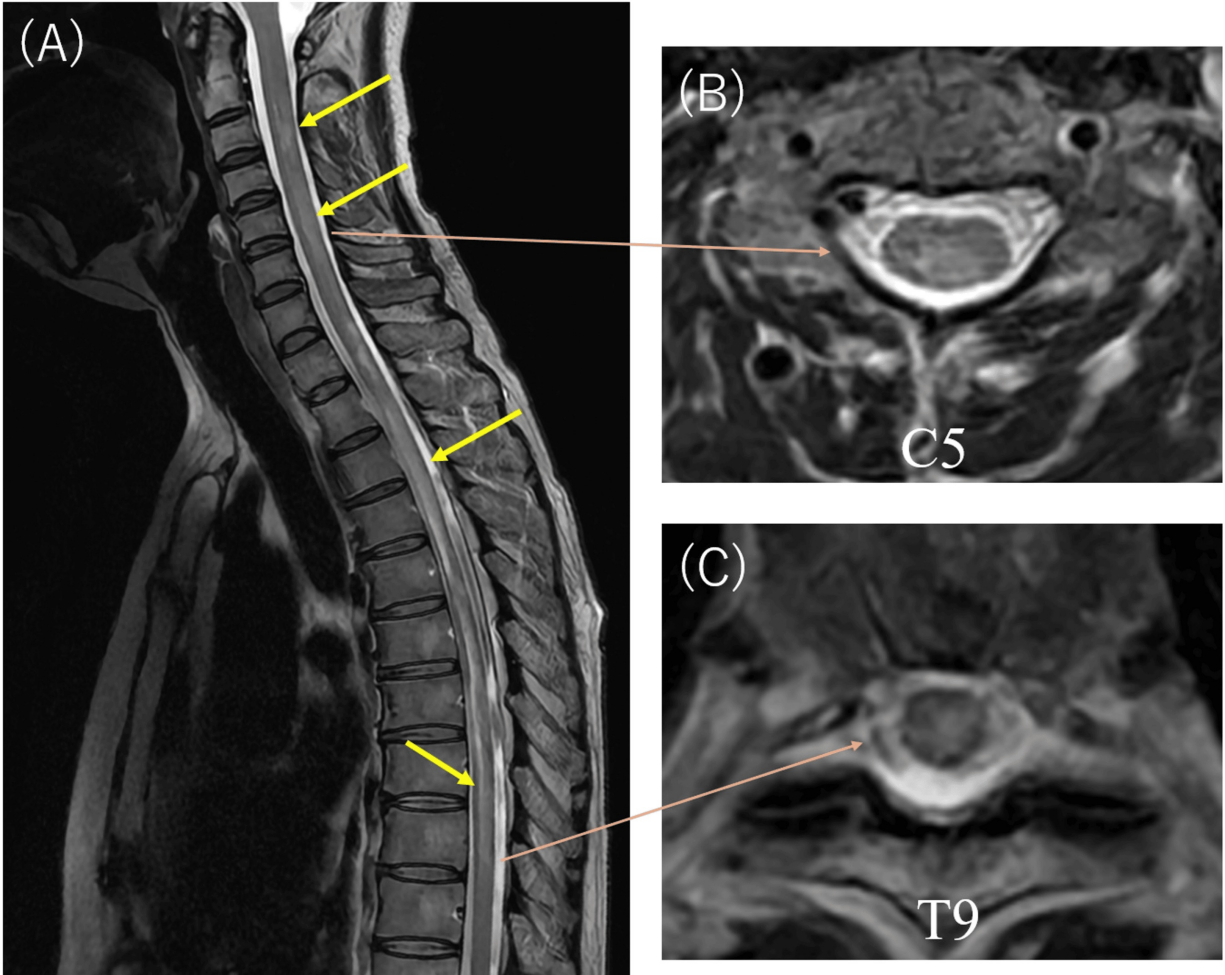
Result of spinal MRI (A) Spinal MRI using T2-weighted, fat-suppressed fast spin echo sequences demonstrates long cord lesions extending from the cervical to the thoracic spinal cord (yellow arrows). (B, C) Axial sections through the cervical and thoracic spinal cord reveal transverse abnormal signals within the cord (C5 and T9 levels shown, orange arrows).
